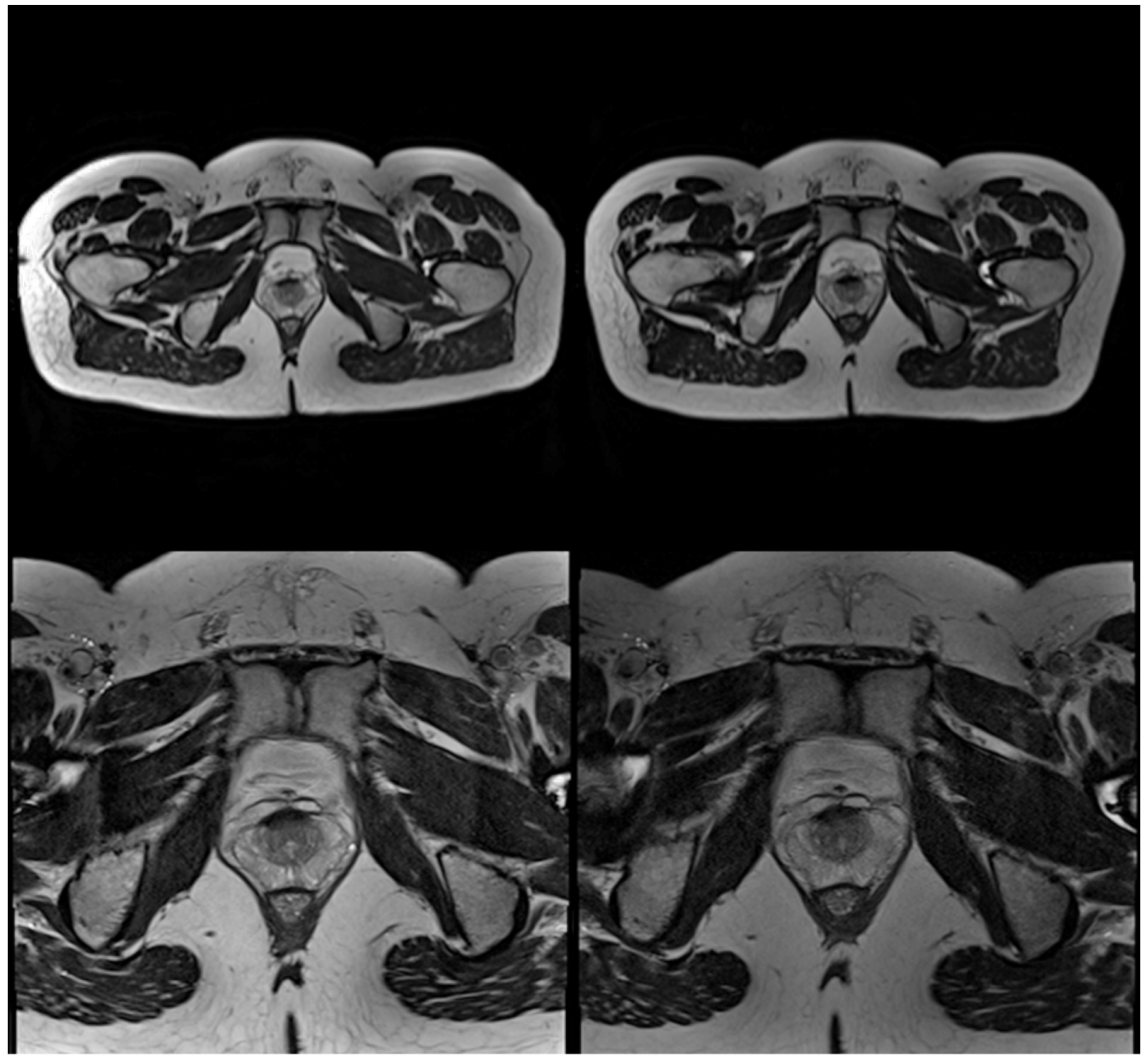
MRI Large field of view (LFOV) (top) and small field of view (SFOV) (bottom) images acquired using conventional scanning protocol, I_standard (left) and radiotherapy‐dedicated protocol, I_MRISim (right). Both images with the same FOV were output with the same window/level setting.
